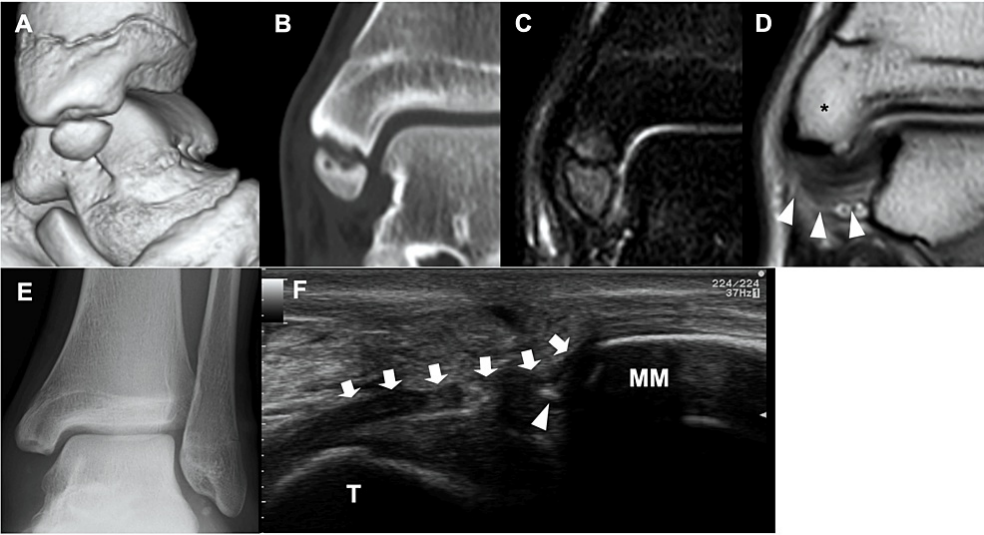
Pre- and postoperative images (A) Three-dimensional computed tomography (CT) shows almost the whole anterior colliculus and intercollicular groove of the medial malleolus is defective and the ossicle corresponds to this defective part. (B) Coronal plane CT reveals that there are subchondral sclerosis, cysts, and irregular cortex on each contact surface between the medial malleolus and ossicle. (C) The T2-weighted fat-suppressed image demonstrates that bone marrow edema is present on the ossicle and corresponding part of the medial malleolus. (D) Most part of the deep posterior tibiotalar ligament (arrowheads) is attached to the posterior colliculus of the medial malleolus (*). (E) Plain radiographs show that the ossicle was resected completely. (F) Postoperative US imaging shows that the superficial component of the deltoid ligament and the deep anterior tibiotalar ligament (arrows) were reattached to the medial malleolus by suture wire (arrowhead). T: talar head
